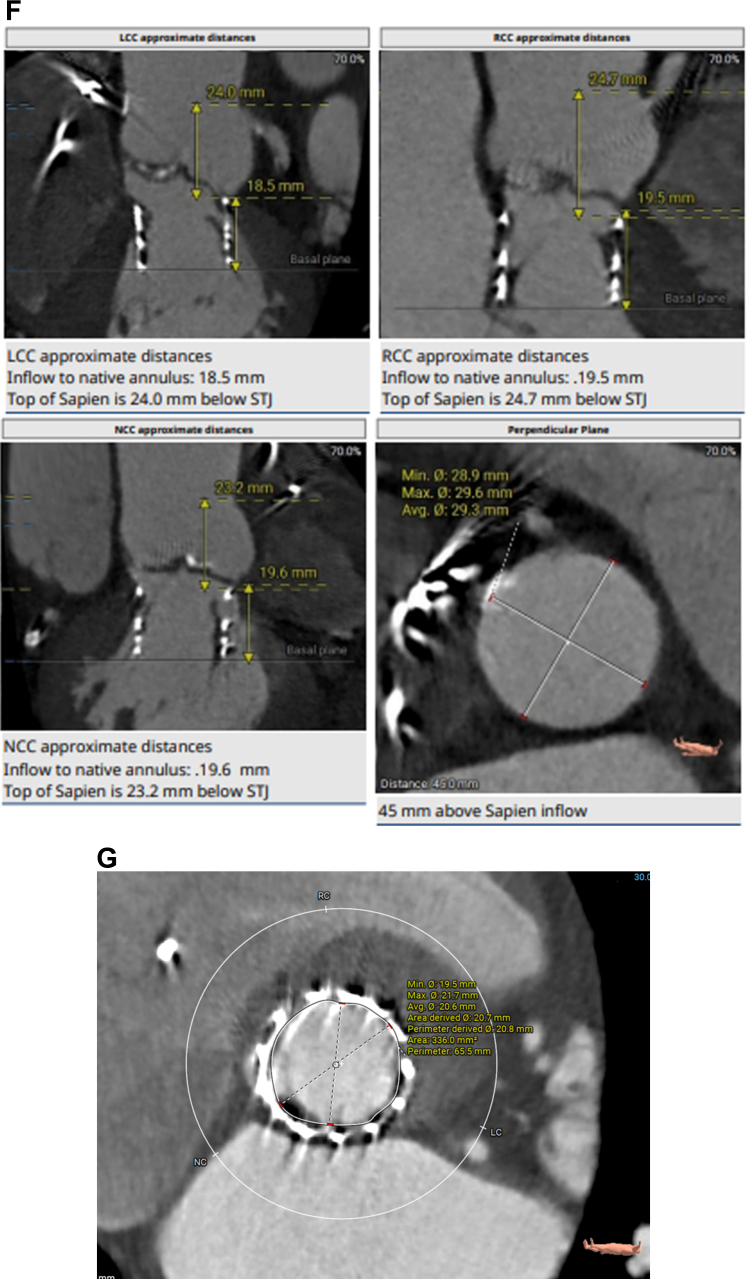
CTA images (A) CW Doppler echocardiogram showing increased gradients across the aortic valve. (B to G) Repeat CTA images after S3 migration into the LVOT below the aortic valve annulus. Abbreviations as in Figure 1.
